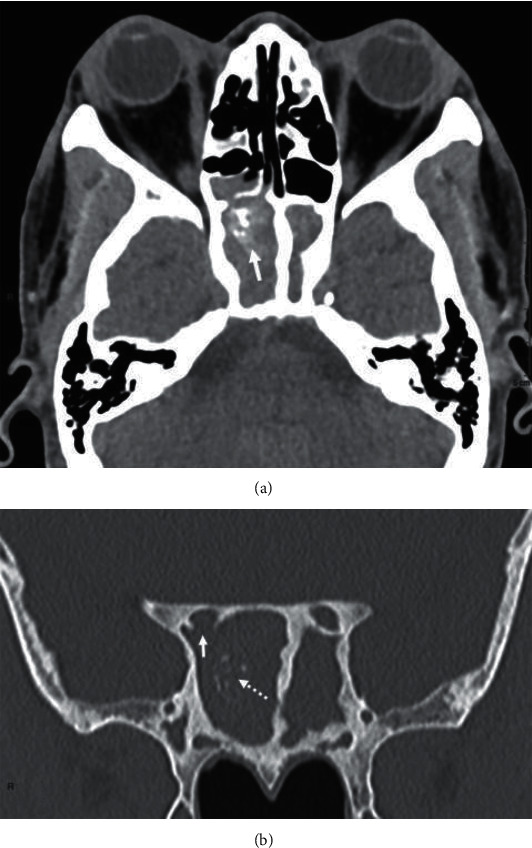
Head computed tomography scan. (a) Unenhanced axial transverse view in parenchymal reconstruction algorithm showing filling of the sphenoid sinus and of the right-sided posterior ethmoid cell by inflammatory material. Observe heterogeneous and amorphous calcium deposits within the right sphenoid cell (arrow). (b) Coronal reformatted view through the sphenoid in bone reconstruction algorithm showing disruption of the floor of the right optic canal (arrow). Observe calcium deposits within the sphenoid sinus (dotted arrow) and bone thickening of the sphenoid walls.
